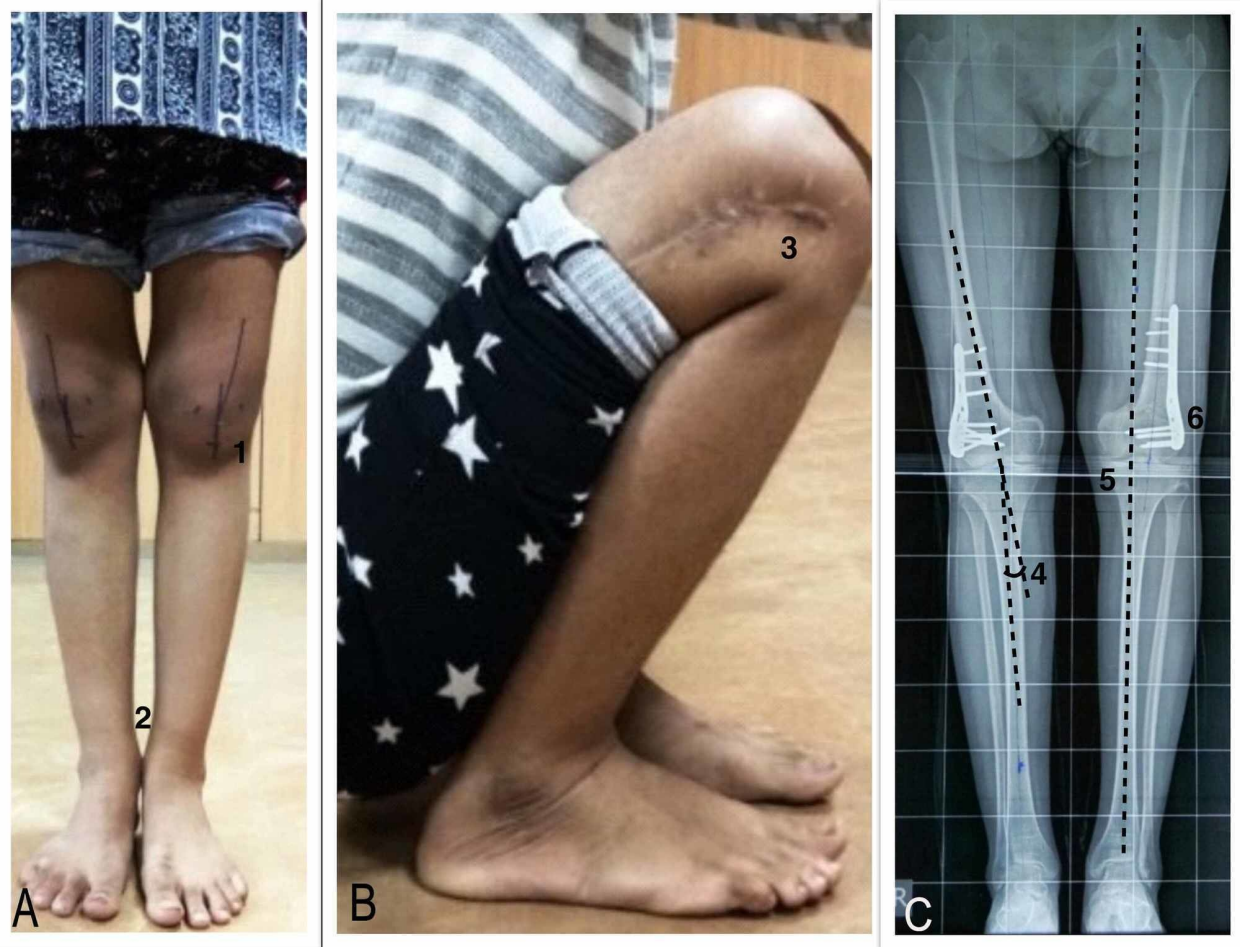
Postoperative clinical and radiographic assessment. A) Measurement of the clinical tibiofemoral angle (1) and the medial malleoli touching each other after the correction of bilateral deformities (2). (B) Full flexion at both knee joints with (3) well-healed surgical scar. (C) Measurement of the radiological tibiofemoral angle (4), mechanical axis passing medial to the tibial spine (5), and 3.5-mm low-profile proximal humeral locking plate (6).
